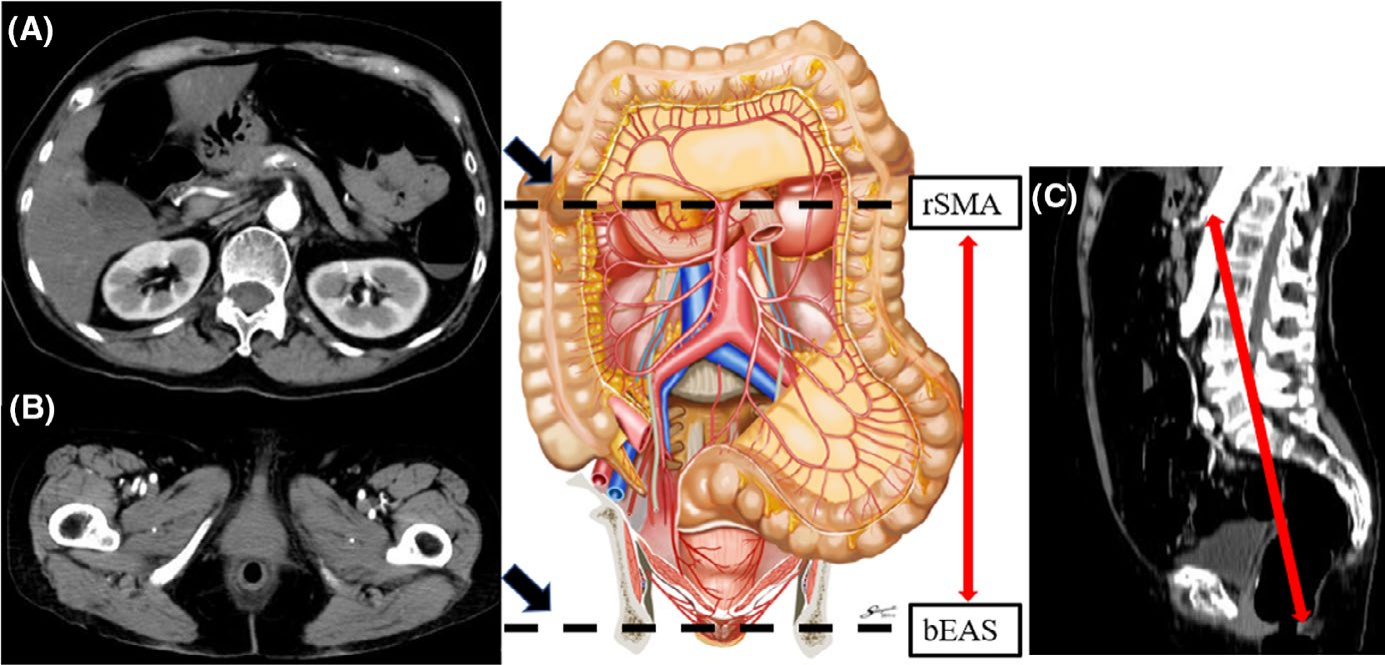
Methods of measuring the distance between the root of the superior mesenteric artery and the bottom of the external anal sphincter (rSMA‐bEAS) on CT images. a: Axial image showing the location of the root of the superior mesenteric artery (rSMA). b: Axial image showing the location of the bottom of the external anal sphincter (bEAS). c: Sagittal image showing the fixed angle such that rSMA and bEAS are on the same slice
